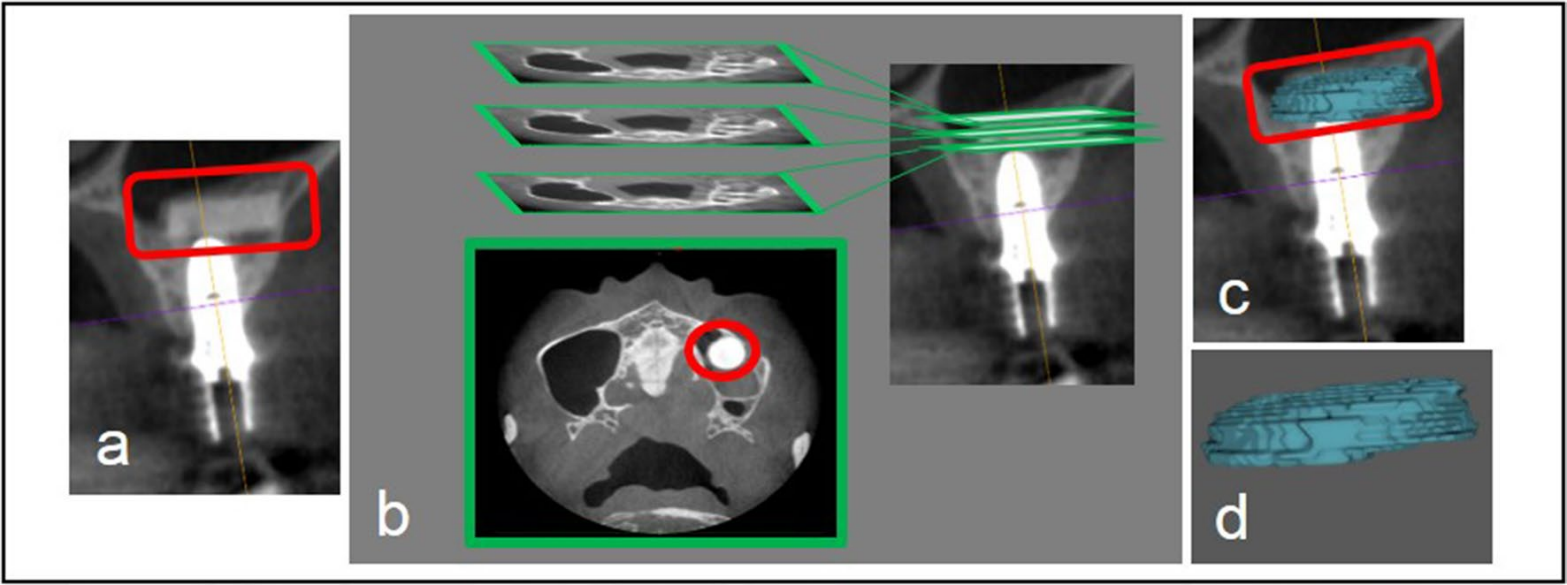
Three-dimensional construction of the TCP plate using axial images from CBCT. A CBCT data with TCP plate in the red frame. b Selection of the borders of the lesion on every slice of the sequence. c, d Creating 3D models of the TCP plate (the TCP plate is in the red frame)
